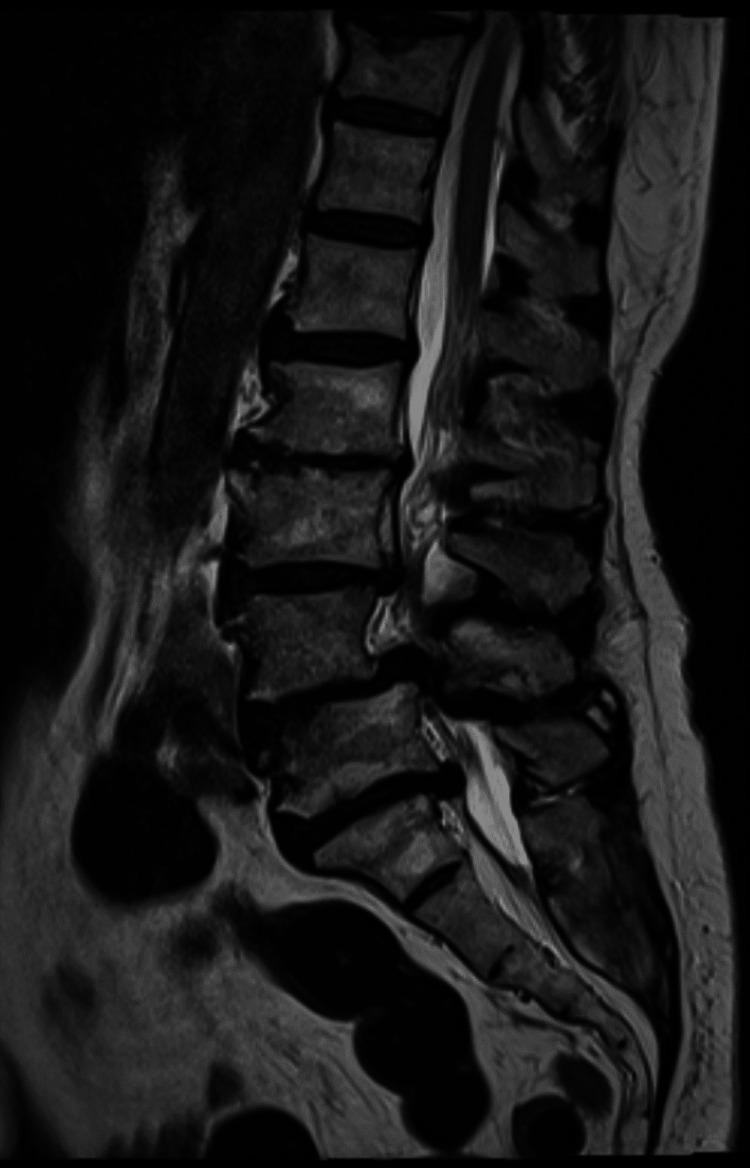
Pre-operative lumbar magnetic resonance imaging
